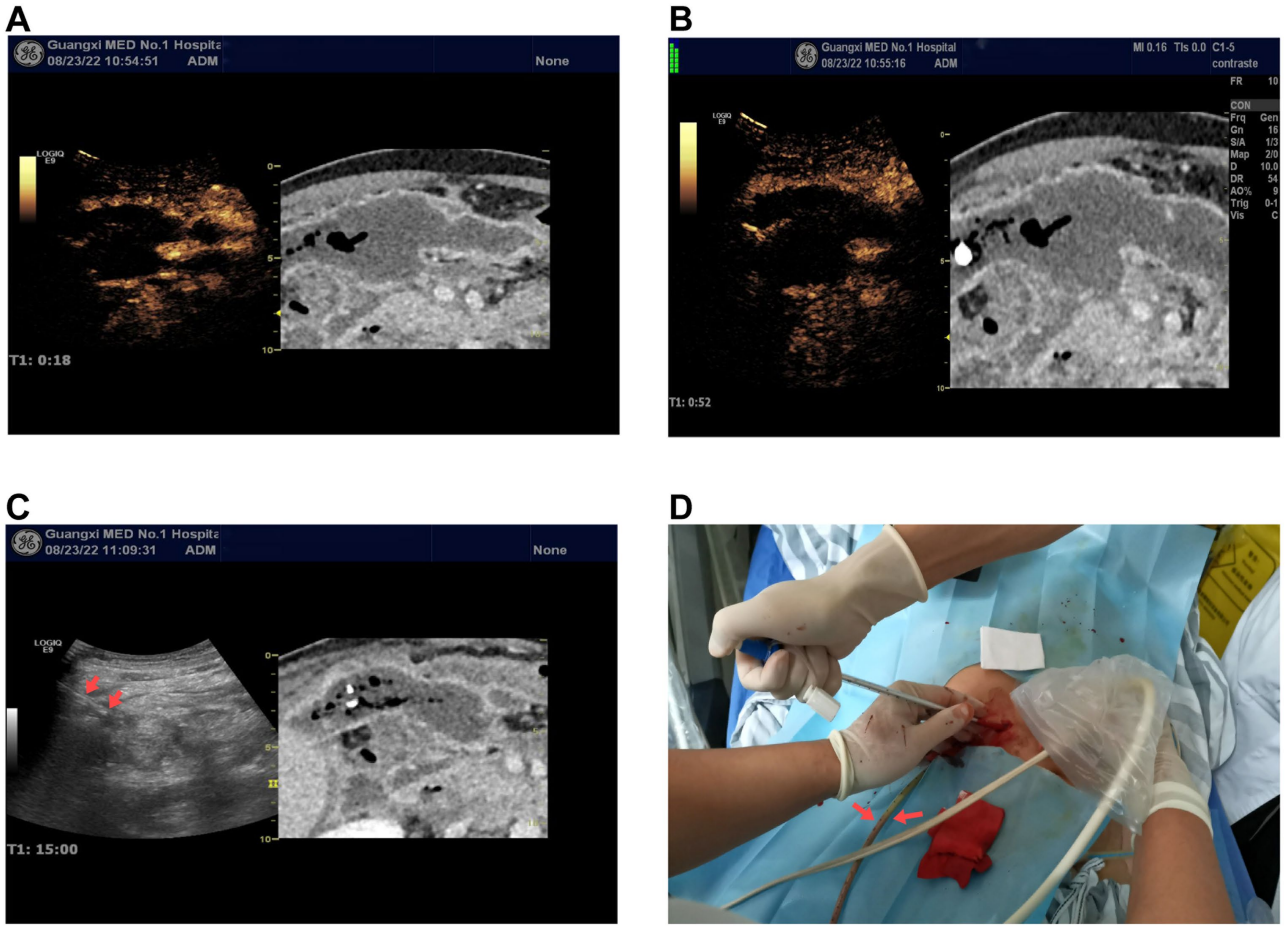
(A,B) Ultrasound contrast-enhanced CT fusion imaging virtual navigation shows early enhancement (18 s) and late enhancement (60s) images of the peripancreatic abscess. The left side of the images shows the ultrasound contrast-enhanced images, while the right side shows the enhanced CT images. Both the ultrasound contrast and enhanced CT images show the peripancreatic abscess as non-enhancing. (C) Percutaneous catheter drainage under ultrasound-CT fusion imaging virtual navigation. The arrow on the left ultrasound image indicates one of the catheters. (D) Intraoperative image of catheter placement as shown in (C), demonstrating purulent fluid draining from one of the catheters.
